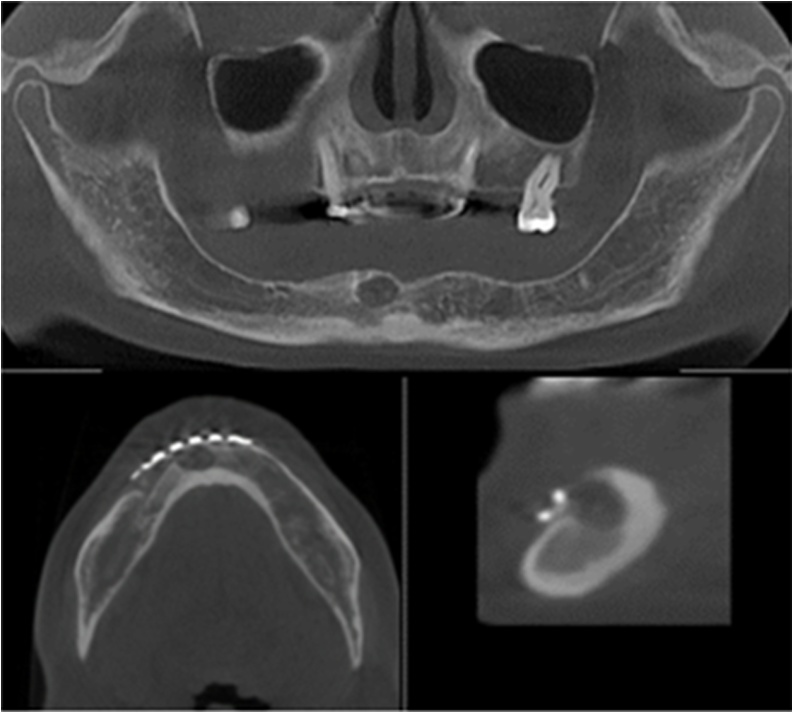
CT images assessment showed areas radiolucent surrounding the region submitted to surgical procedure.
